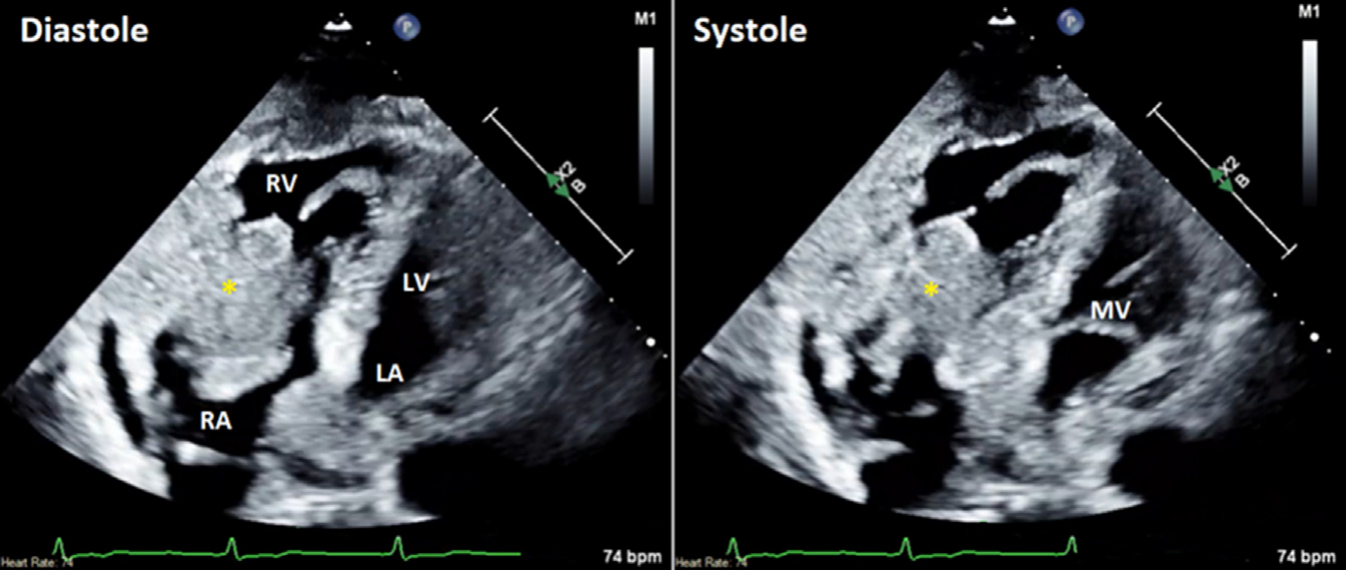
Transthoracic Echocardiogram (Subcostal 4-Chamber View) The yellow asterisk denotes a large tumorlike mass with subtotal tricuspid obstruction. LA = left atrium; LV = left ventricle; MV = mitral valve; RA = right atrium; RV = right ventricle.
